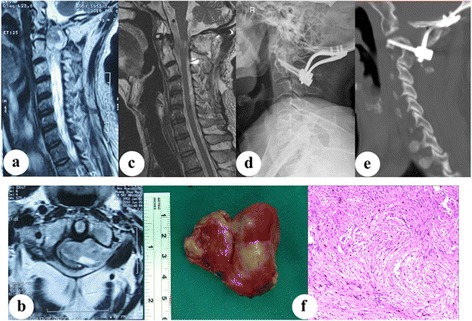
Case 5. This 58-year-old male patient experienced a significant deterioration for his neurological condition several months and presented with neck pain and dysesthesia and was unable to walk properly. a preoperative sagittal T2-weighted MR image: spinal extramedullary tumor locates anterior to the spinal cord in the craniocervical region. b preoperative axial T2-weighted MR image: this lesion leads to destruction of the C1 posterior arch. c postoperative sagittal T2-weighted MR image: the tumor has been removed completely. d postoperative X-ray: occiput-C2 fixation has been used by cervical pedicle screws and occipito-cervical plates. e CT images at the final follow-up: the success of spinal fusion using autologous iliac bone. f postoperative photograph: a well-encapsulated tumor is more than 5 cm across, and pathology report confirms the diagnosis of schwannoma
